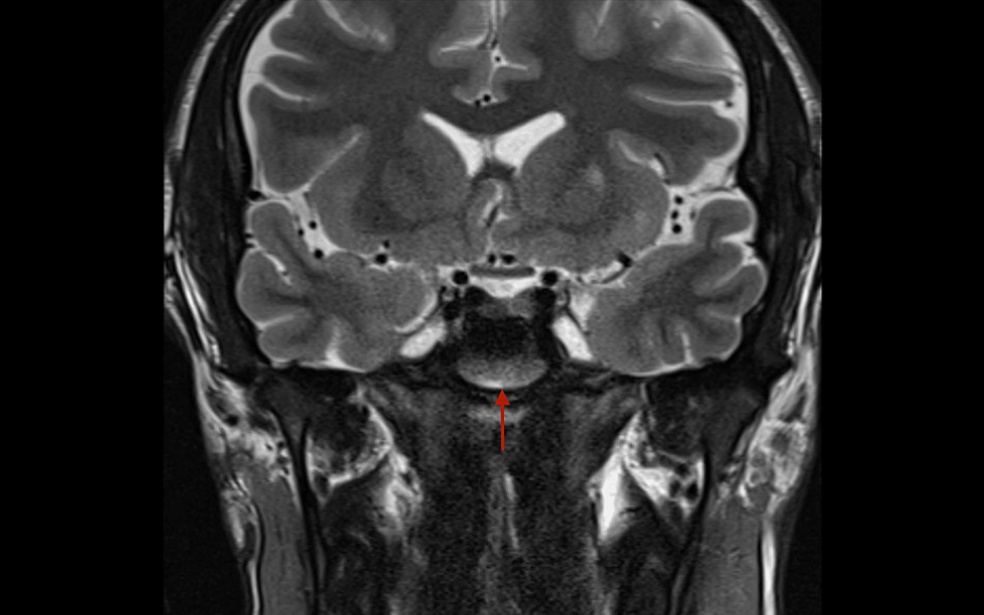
MRI head showing faint intertriginous hypo-enhancing area in the pituitary.
